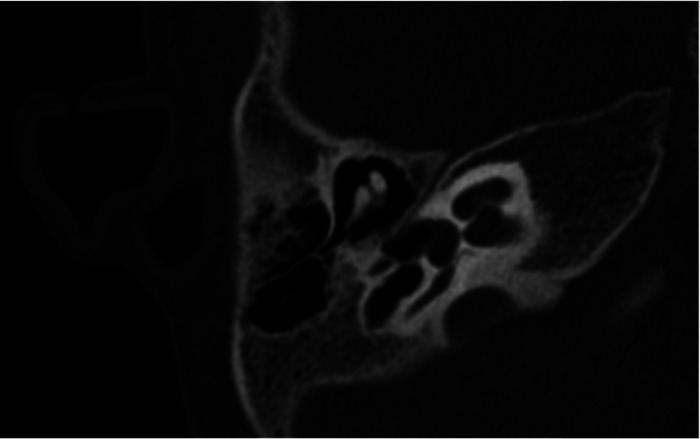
CT scan of a narrow fallopian canal of the facial nerve.
